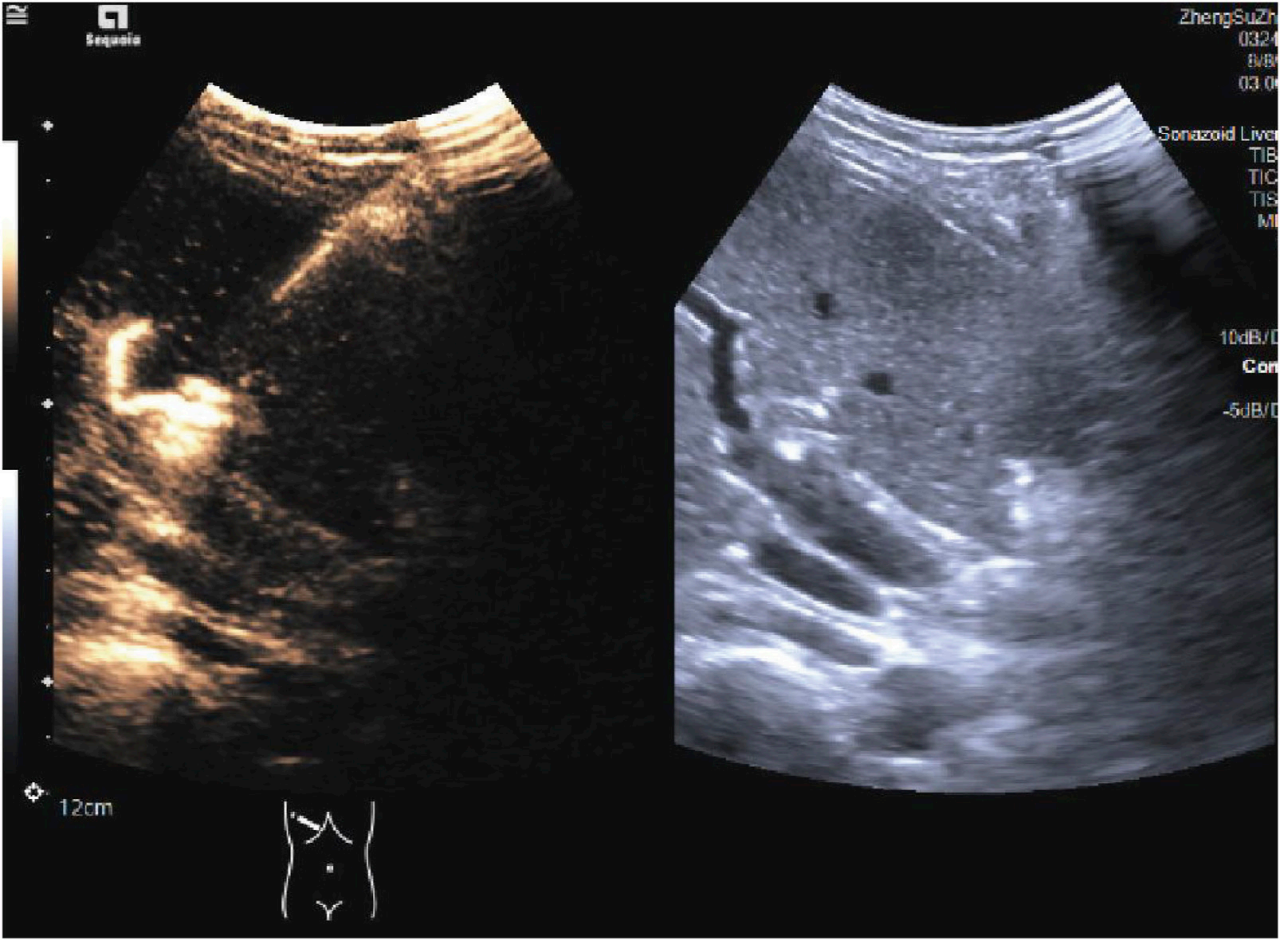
Ultrasound imaging of Glisson’s capsule after the administration of ultrasound-guided HHNB in dual-screen Doppler mode. HHNB: hepatic hilar nerve block.
